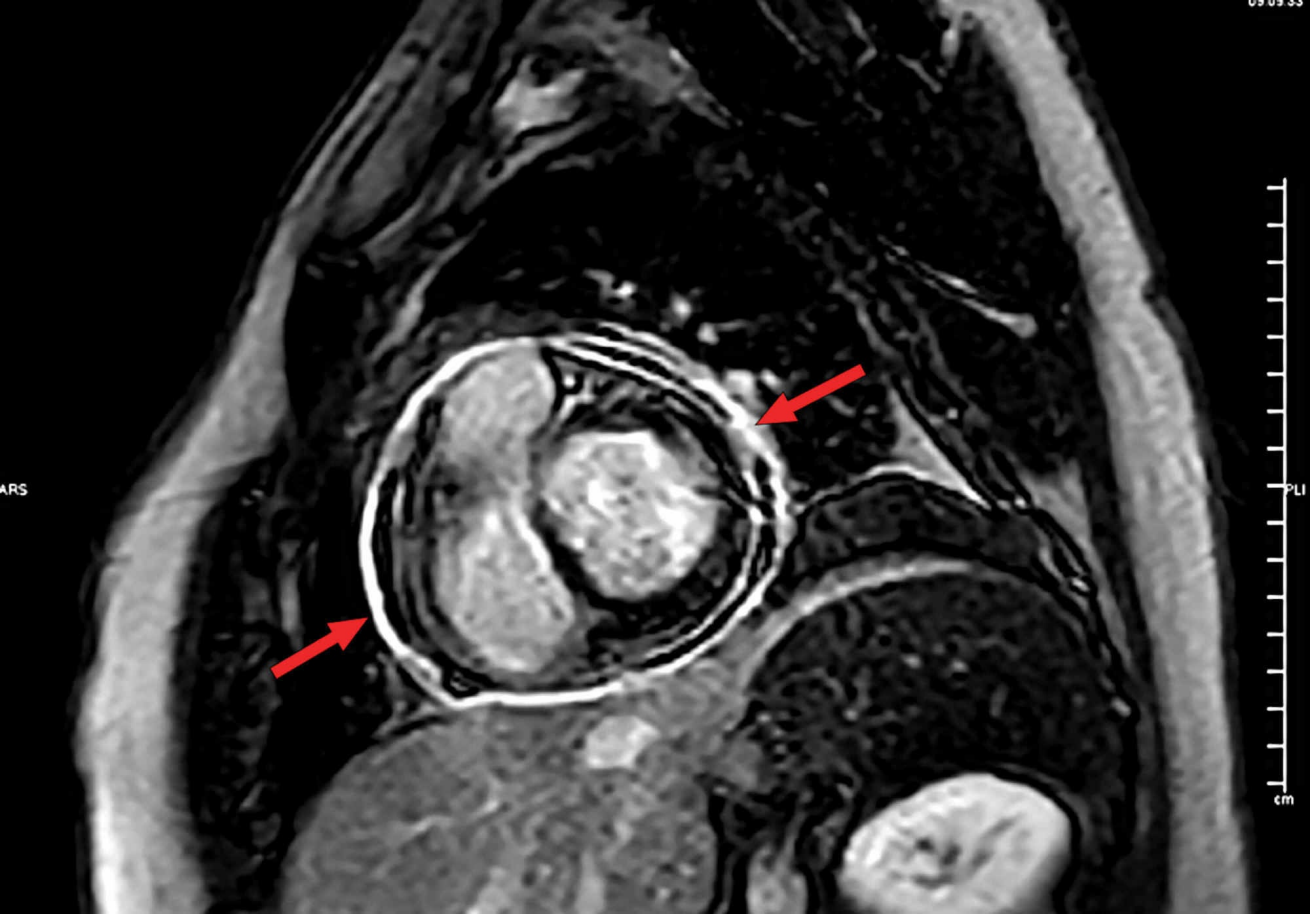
MRI short-axis view. Red arrow: septal bounce (late gadolinium enhancement).
